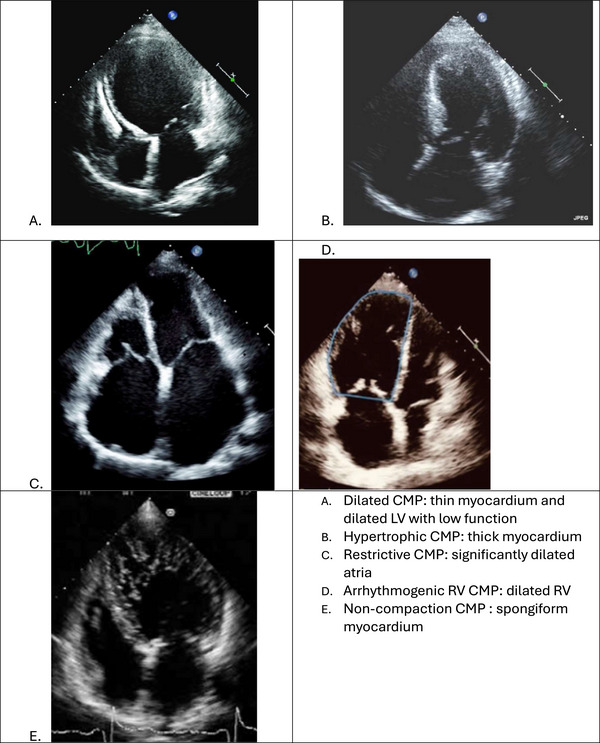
Major cardiomyopathies and their main echocardiographic features. (A) Dilated CMP: thin myocardium and dilated LV with low function. (B) Hypertrophic CMP: thick myocardium. (C) Restrictive CMP: significantly dilated atria. (D) Arrhythmogenic RV CMP: dilated RV. (E) Non‐compaction CMP: spongiform myocardium.
